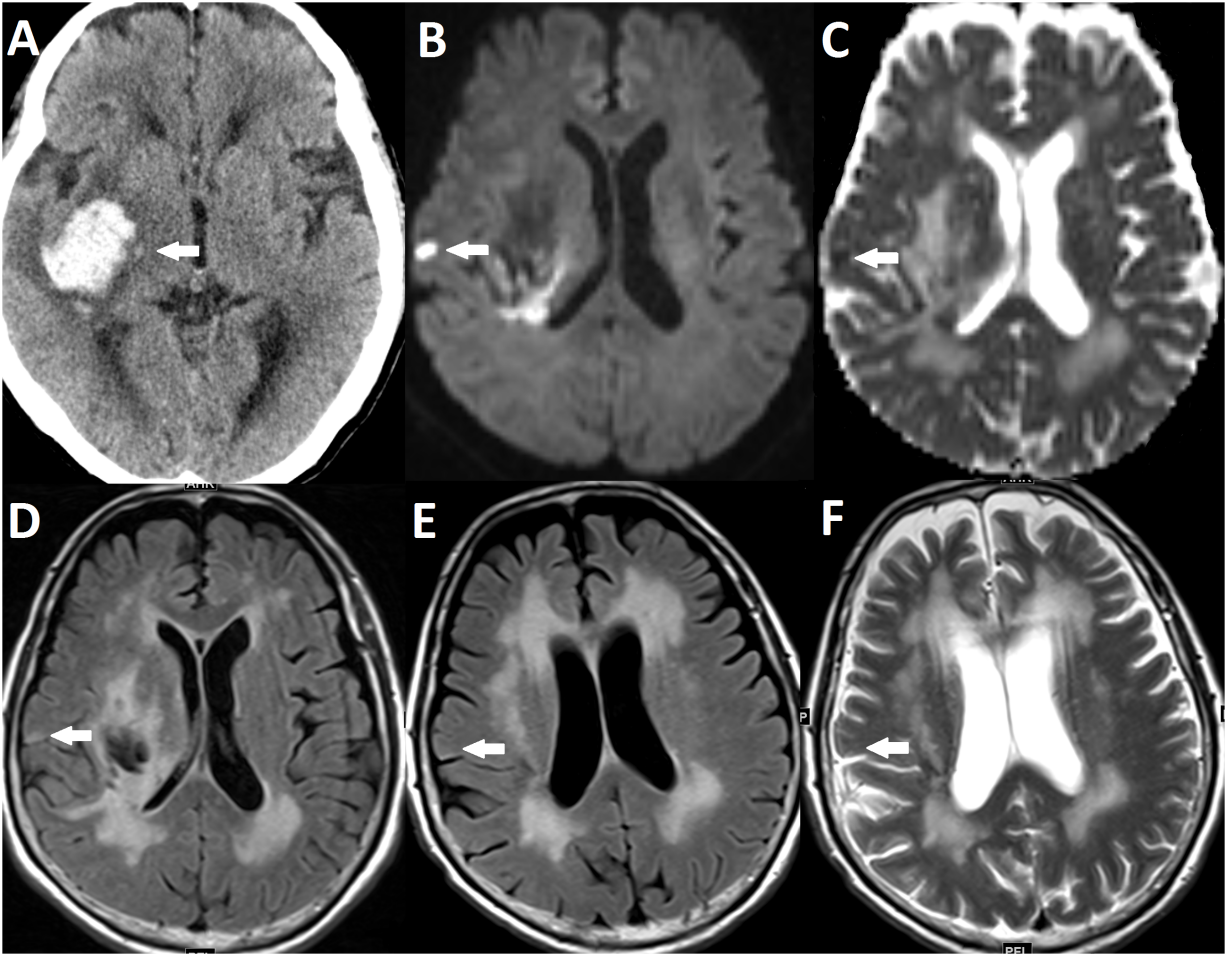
Example of a DWI lesion not visible on follow-up MRI. (A) CT showing ICH in right putamen (arrow). (B, C and D) Baseline MRI showing a DWI lesion that has high signal intensity on DWI (B), low signal intensity on ADC (C) and equivocal high to intermediate signal intensity on FLAIR (D). (E and F) The DWI lesion is not visible on FLAIR (E) and T2WI (F) MRI 3 months after ICH.
